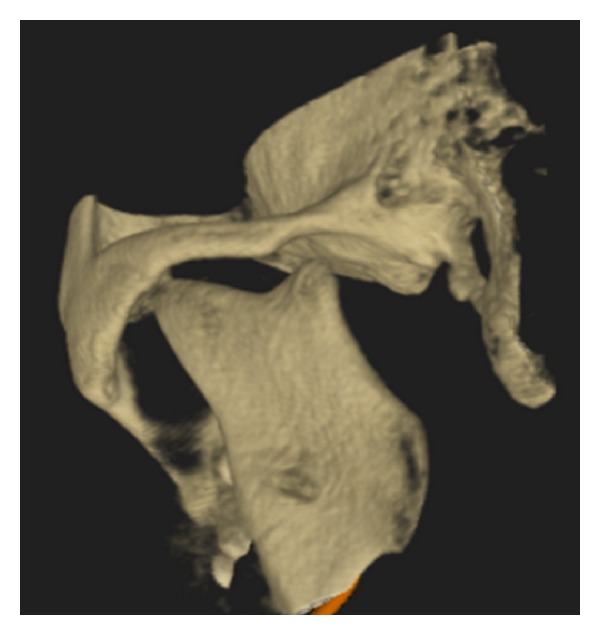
3D CBCT left oblique lateral—shows hypoplasia of glenoid fossa and condyle.
